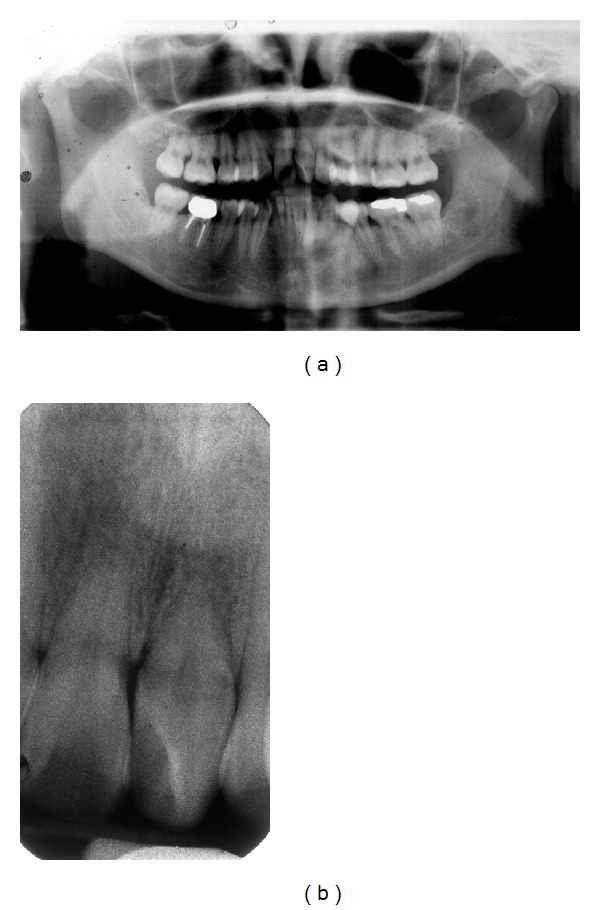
Pretreatment panoramic and periapical radiographs.
